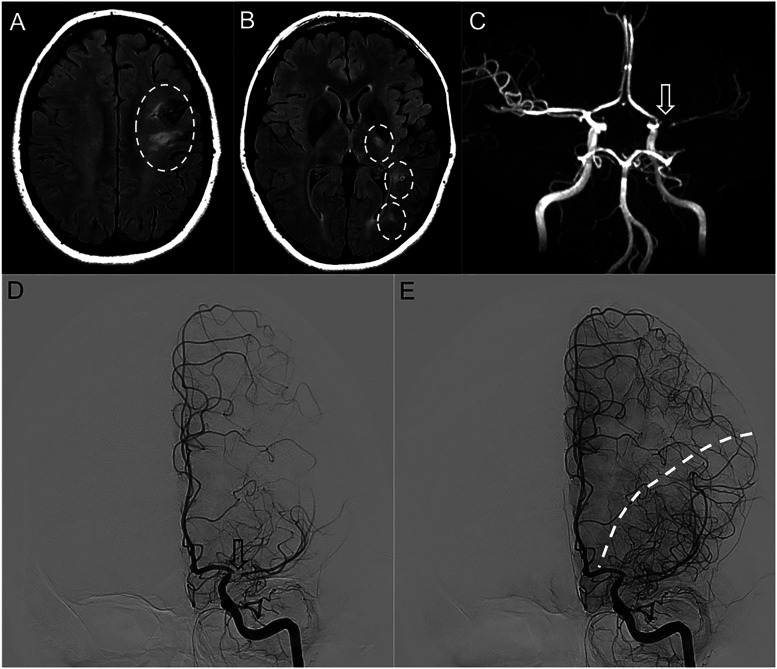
Axial T2-FLAIR images demonstrated areas of hyperintensity and gliosis in the left frontal lobe (a), left corticospinal tract and left optic radiation (b). MRA-TOF imaging (c) demonstrated high-degree stenosis of the left proximal M1 segment (arrow). Digital subtraction angiography (DSA) demonstrating left ICA injections in the early arterial (d) and late arterial (e) phases, confirming the steno-occlusive segment of the proximal left M1 segment (arrow). Late arterial phase showed a shift of the watershed towards the 3 o’clock position (dashed line), with extensive collaterals from the left ACA.
